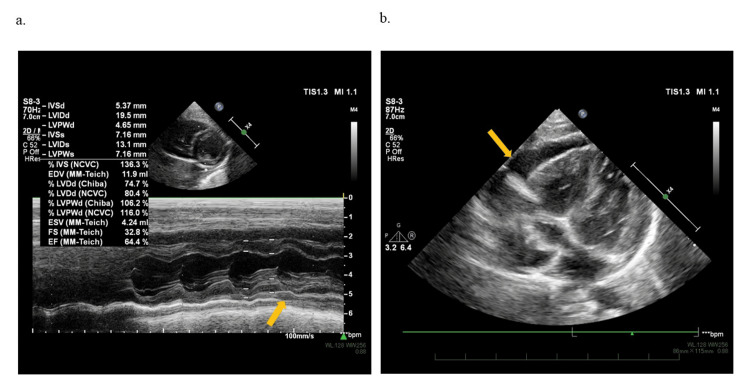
Two-dimensional echocardiography findings (a) Normal left ventricular ejection fraction throughout the cardiac cycle, with evidence of pericardial effusion. (b) Pericardial effusion measuring up to 6.6 mm visible in front of the right atrium and right ventricle
